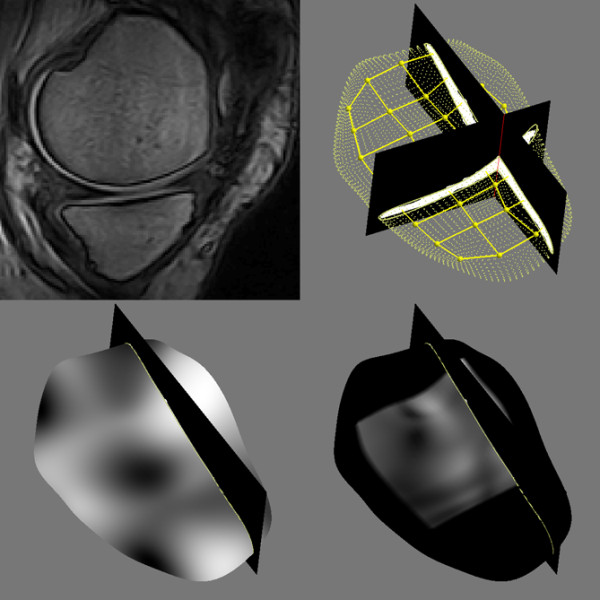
Magnetic resonance imaging-based biomarker quantification framework. Top left: a slice from a magnetic resonance imaging scan. Top right: segmentation of the medial tibial cartilage compartment shown in sagittal and coronal slice with a shape model fitted to the segmentation. Bottom left: thickness map. Bottom right: curvature map in the central region of interest used for the curvature marker. All computational steps are fully automatic.
